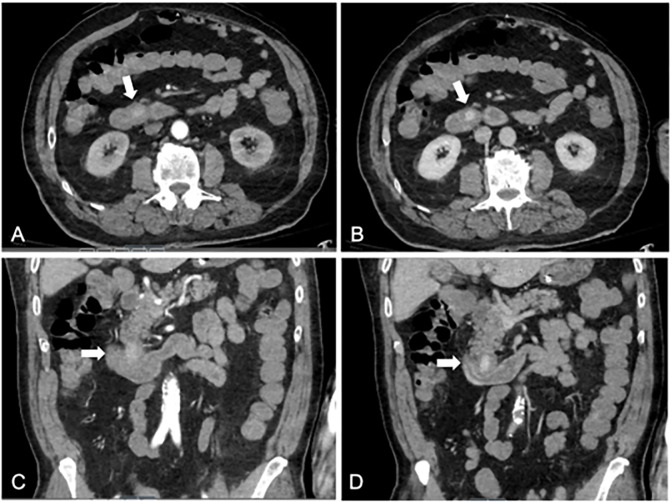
Computed tomography angiogram of abdomen showing submucosal duodenal pseudoaneurysm (white arrows) causing massive gastrointestinal hemorrhage in (A) transverse plane arterial phase, (B) transverse plane venous phase, (C) coronal plane arterial phase, and (D) coronal plane venous phase.
